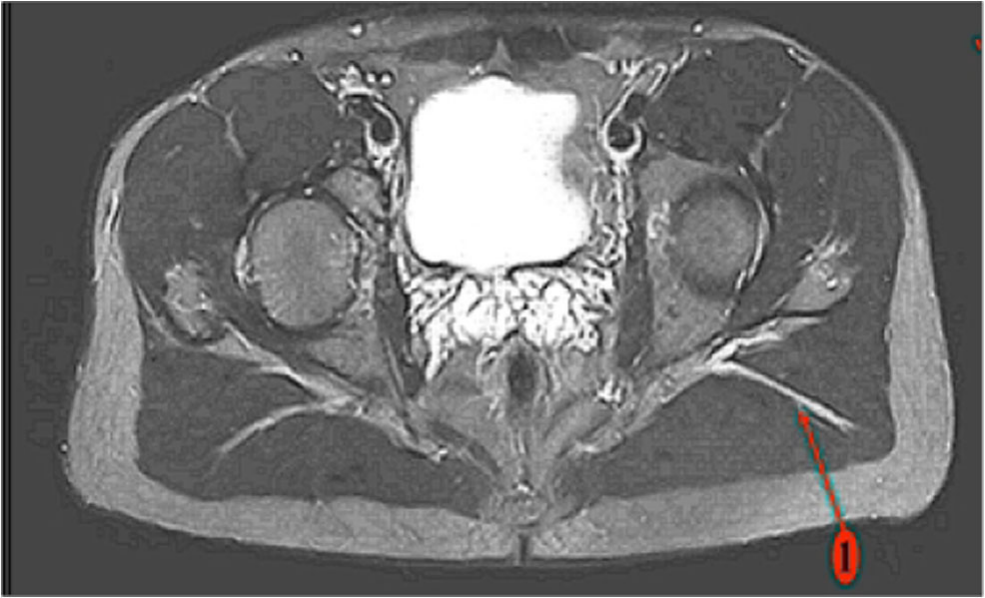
Posterior and anterior heads of the gluteus maximus (spot 1) (MRI image)
